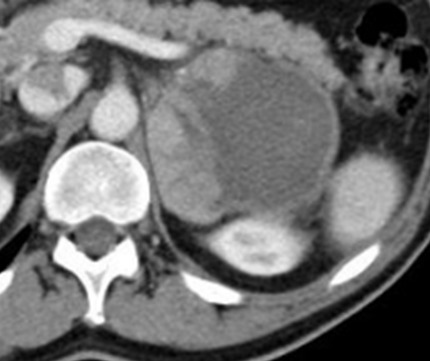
Abdominal CT scan showing left adrenal tumour of 9 cm
